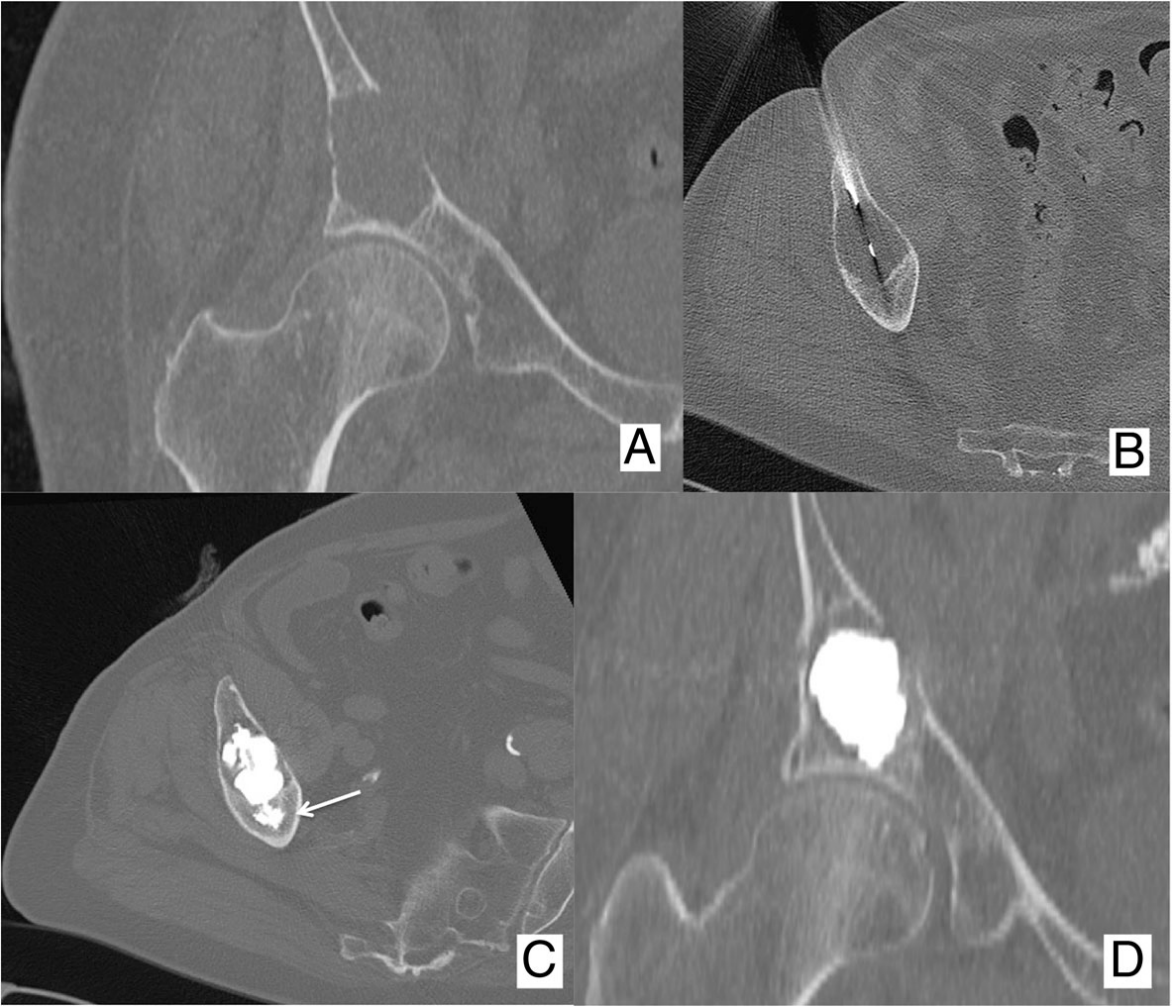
Eighty-five-year-old male patient presenting with an acute mechanic pain of the right hip. a A CT scan revealed a large lytic lesion of the acetabulum without any sign of pathologic fracture. The patient underwent (b) percutaneous biopsy that revealed a metastasis from kidney cancer; c, d in the same session, the patient received osteoplasty with fast and effective pain relief. Of note, PMMA was anchored in the distal normal bone (arrow) before filling the lytic cavity
